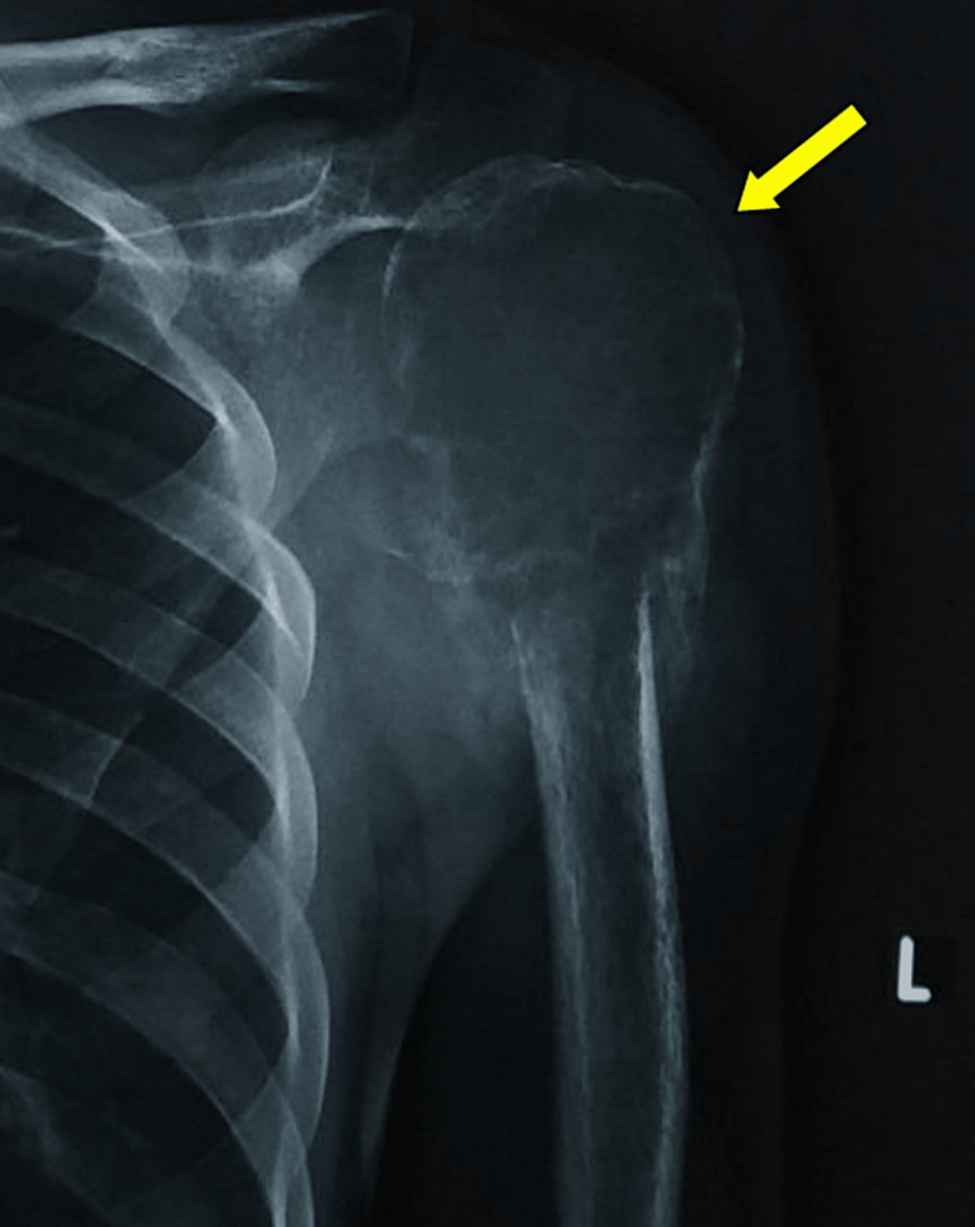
A plain radiograph at the index visit
